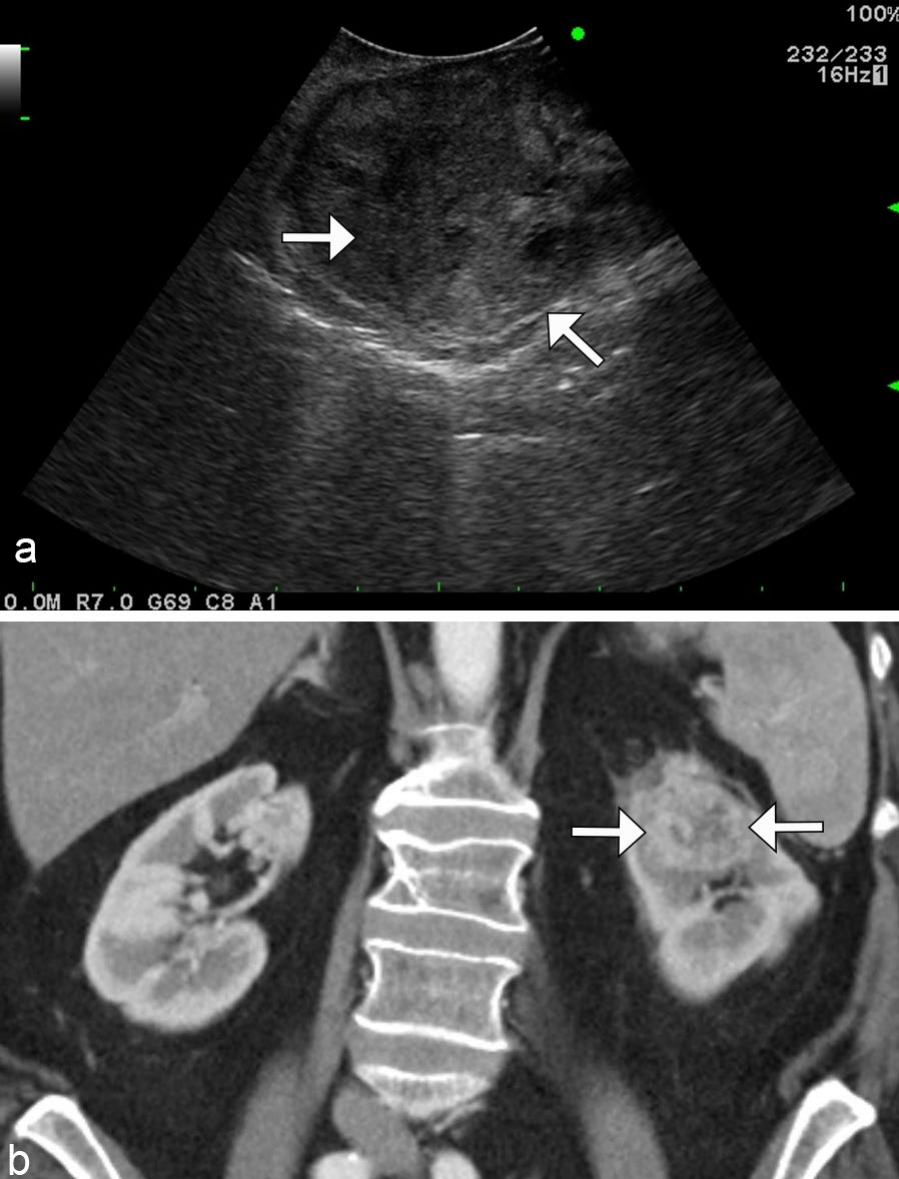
Clear cell renal cell carcinoma.* Sagittal grayscale ultrasound (a) demonstrates a heterogeneous right upper pole renal mass (arrows). Coronal contrast CT (b) demonstrates enhancement of the renal lesion (arrows). Biopsy confirmed renal cell carcinoma. Note the increased vascularity and heterogeneity relative to what would typically be seen in a solitary renal lymphomatous lesion
